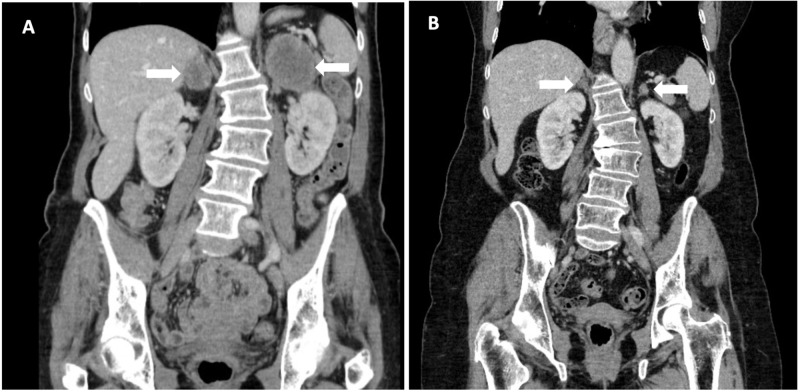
Treatment response with nivolumab A: CT abdomen before initiation of nivolumab demonstrating 6.0 x 4.4cm left adrenal mass and 3.1 x 2.5 cm right adrenal mass. B: Near complete resolution of bilateral adrenal masses after 41 months of nivolumab.
